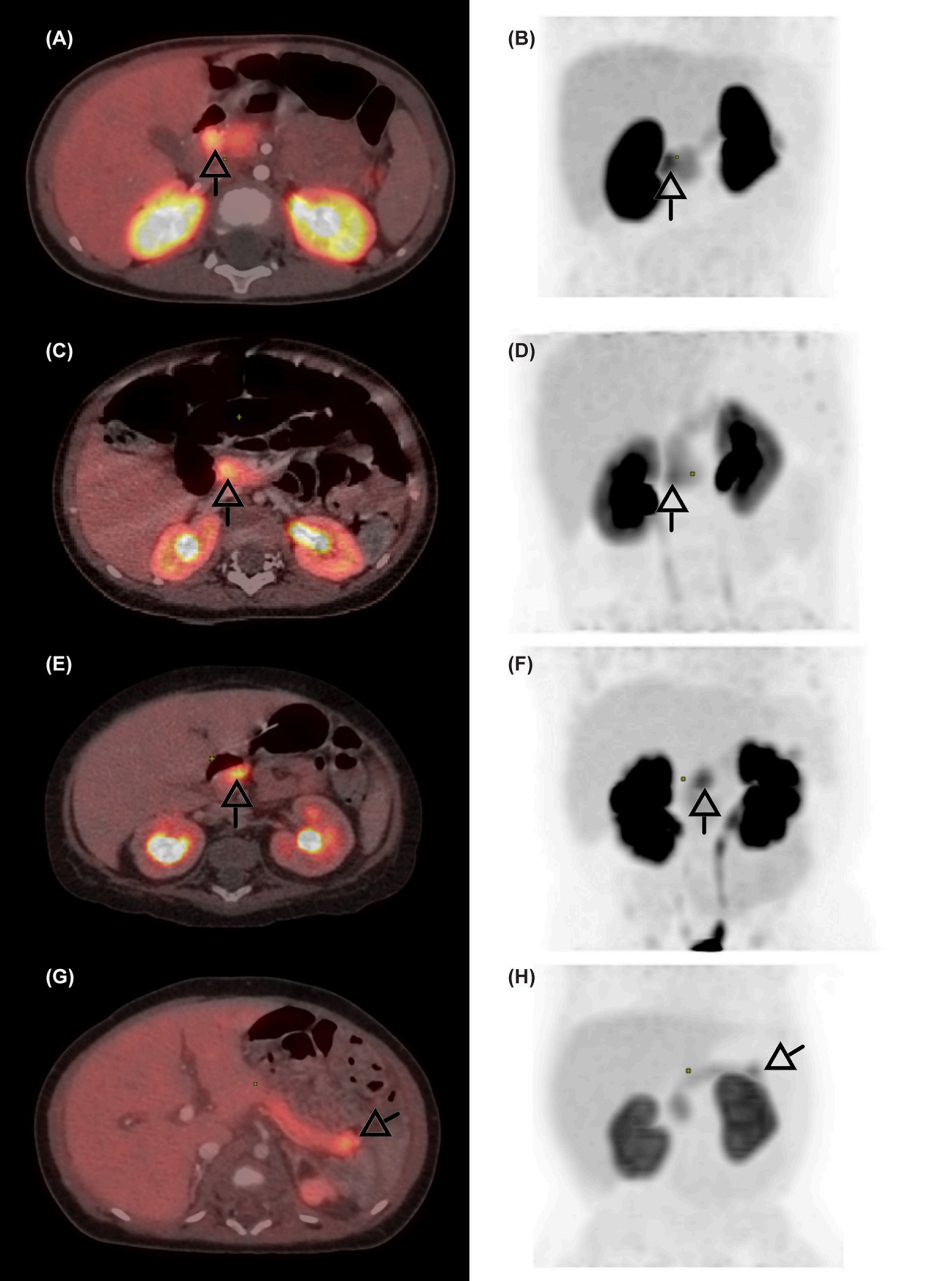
^18^F-DOPA-PET/CT scans of four patients with focal CHI. The focal lesions are seen in the duodenum (A,B), the pancreatic head (C,D), body (E,F), and tail (G,H). Arrows indicate the focal lesion.
